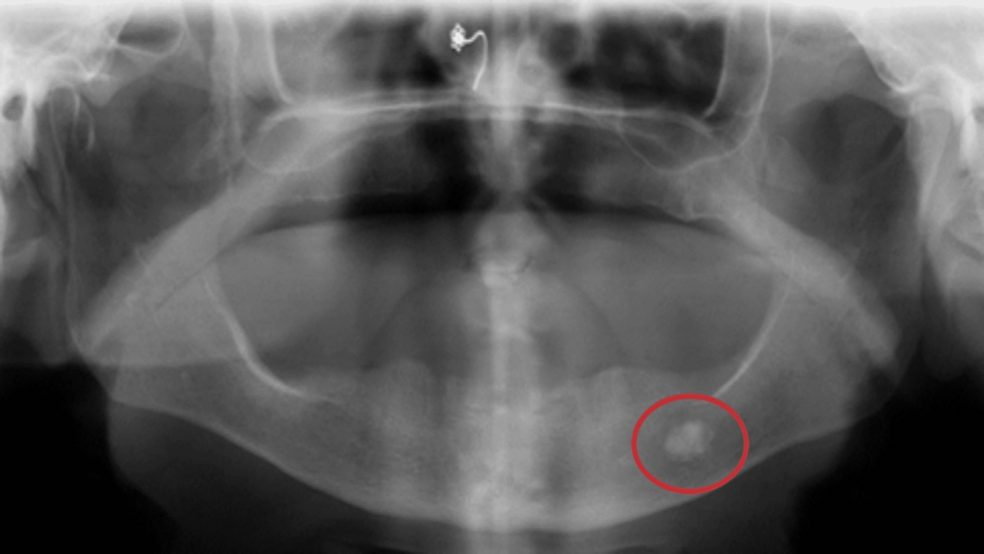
Radiopaque lesion as seen on a panoramic radiograph
